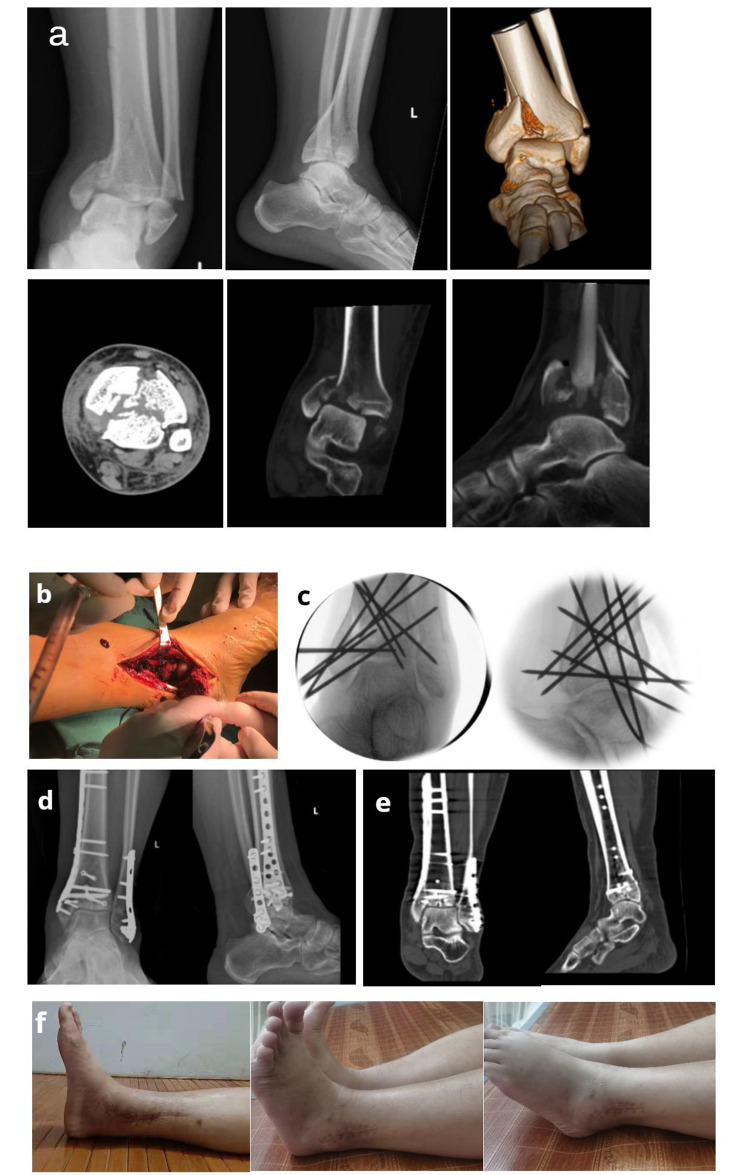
Photographs and radiographs of a 35-year-old male who experienced a varus-type tibial pilon fracture (AO/OTA type C3). (a) Preoperative X-ray radiographs and CT scans showed a varus-type pilon fracture characterized by compression of the medial column of the distal plafond. (b) Intraoperative medial malleolar window approach application (c) Intraoperative radiographs of K-wires temporary fixation technique. (d ~ e) Postoperative X-ray and CT examination showed a satisfactory reduction of the fracture. (f) Functional outcome
